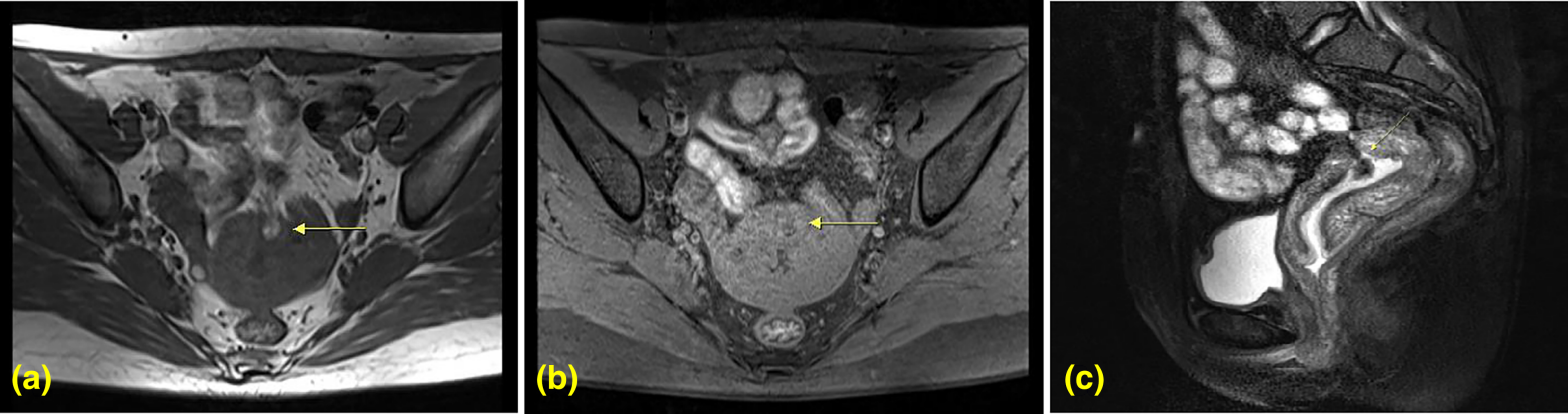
Pelvic magnetic resonance imaging (MRI) showed that adipose tissue was embedded in the anterior wall of the uterus to the uterine cavity by axial T2‐weighted image (a), axial fat‐suppressed T1‐weighted image (b), and sagittal fat‐suppressed T2‐weighted image (c).
